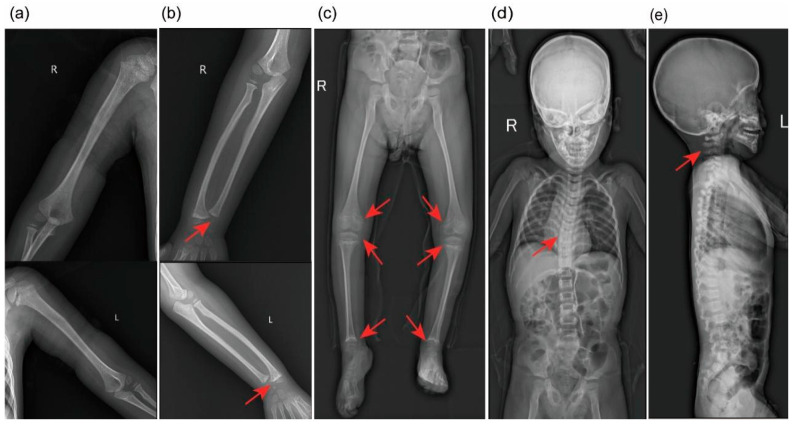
X-rays of the limbs and spine. (a–c) Metaphyseal lesions of the limbs. (d,e) Straightening of the cervical spine curvature and thoracic scoliosis.
